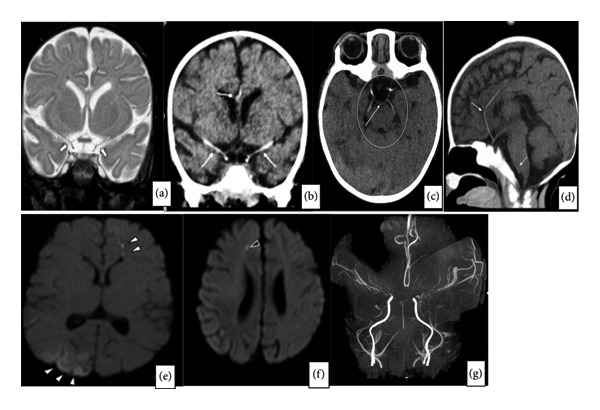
(a–g) Computed tomography (CT) of the head and magnetic resonance imaging (MRI) of the brain in our patient.
